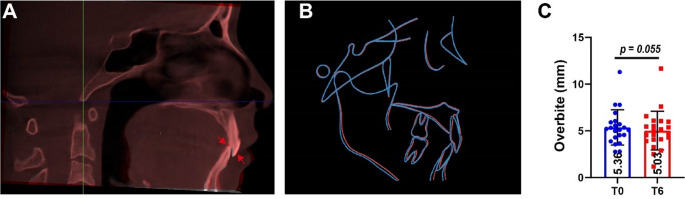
Overbite changes based on cone-beam computed tomography (CBCT) superimposition and measurements. Representative images. (A) Superimposed CBCT images at T0 (red) and T6 (white) after head orientation calibration in Dolphin software. (B) Lateral cephalograms reconstructed from CBCT at T0 and T6 with anatomical outlines. The red outline corresponds to T0, and the blue outline to T6, high lighting overbite changes of the central incisors. (C) Quantitative changes in overbite measurement (paired t-test)
